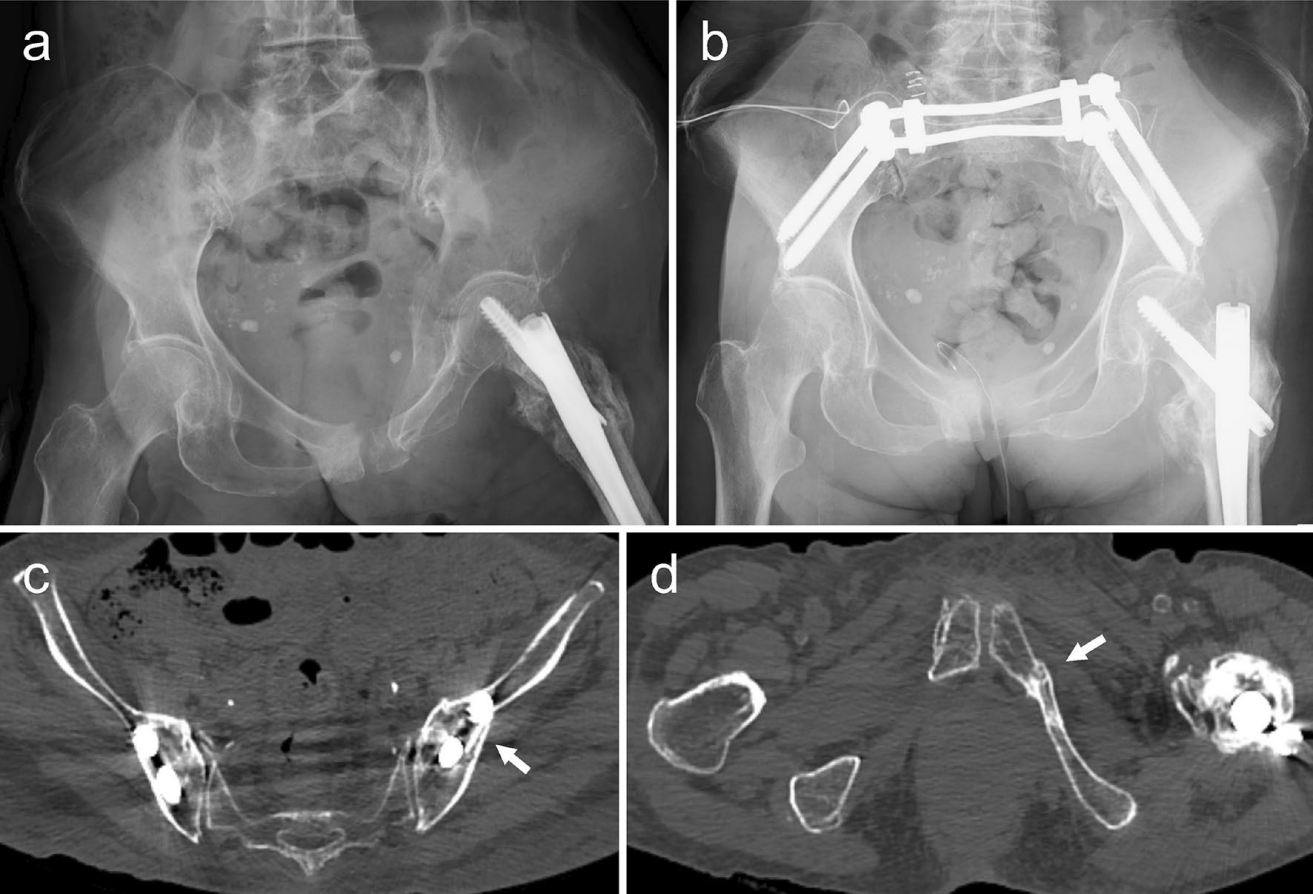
Representative plain radiographs and post-operative computed tomography images. A plain radiograph obtained after the injury (a). A plain radiograph obtained after treatment shows that reduction has been achieved. The obturator foramen appears almost symmetrical (b). Bone union of the left iliac bone (c). Bone union of the inferior ramus of the left pubis (d).
